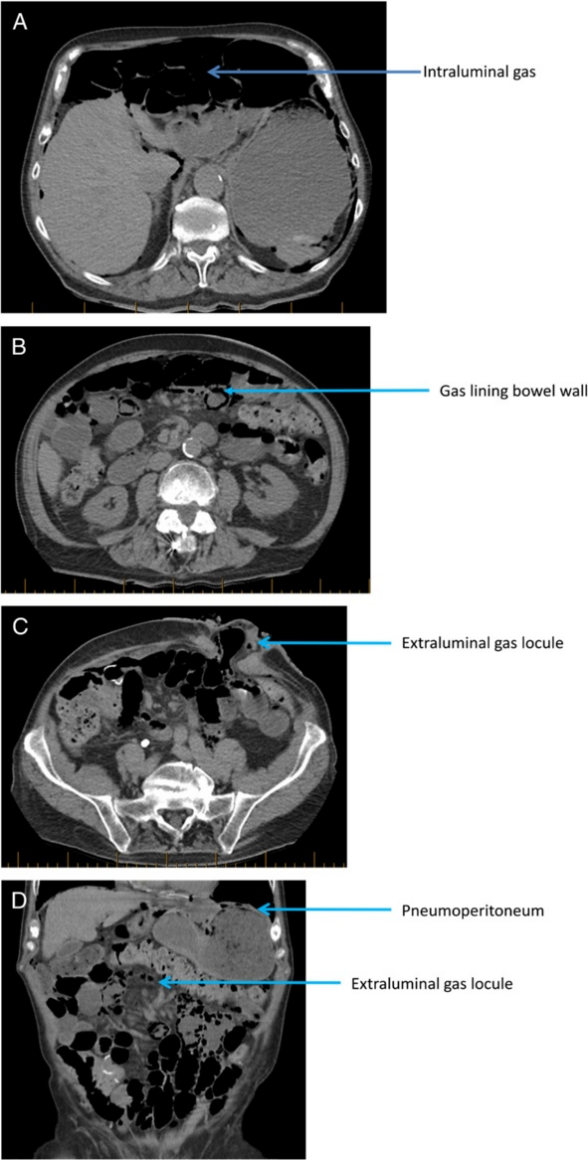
CT scan shows diffuse intramural gas with suspected pneumoperitoneum. a Collection of intaluminal gas in PCI appearing like pneumoperitoneum. b Gas lining the bowel wall. c PCI appearing like extraluminal gas locules. d PCI appering like extraluminal gas locules and pneumoperitoneum
